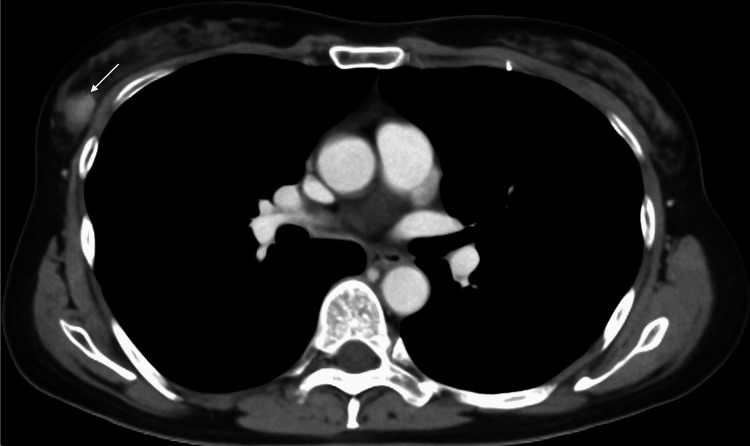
CT findings CT showed a well-enhanced oval mass with circumscribed margins in the right mammary gland (arrow). CT: computed tomography
